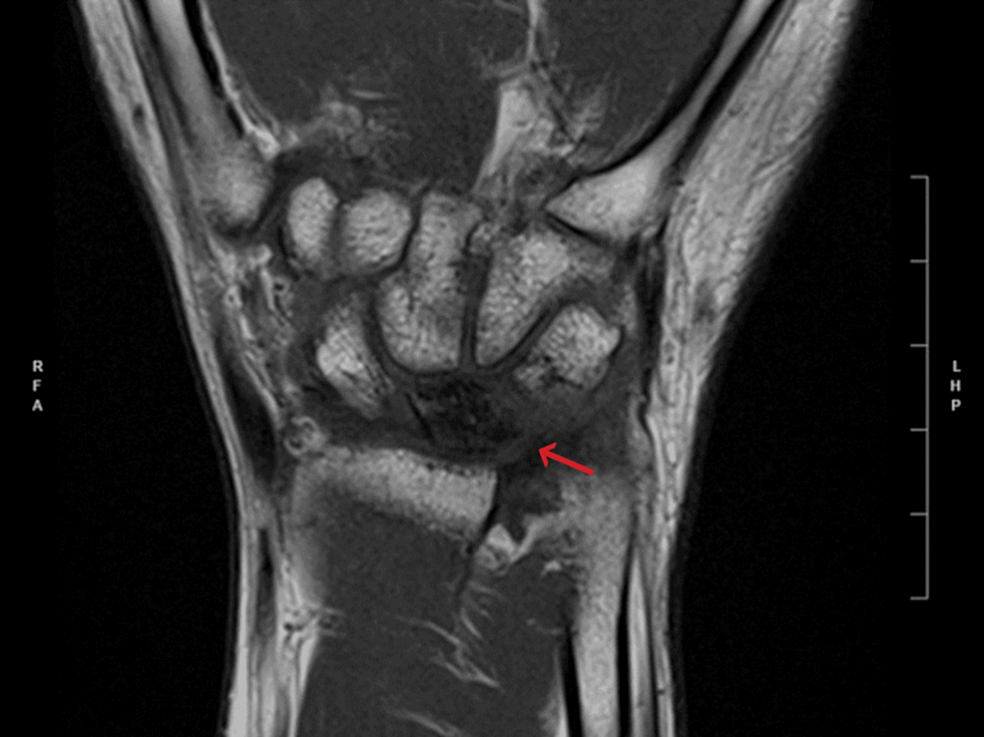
T1 coronal MRI The arrow corresponds to a diffuse low signal in the lunate bone. Image #10/19; spin echo (SE): 10001
